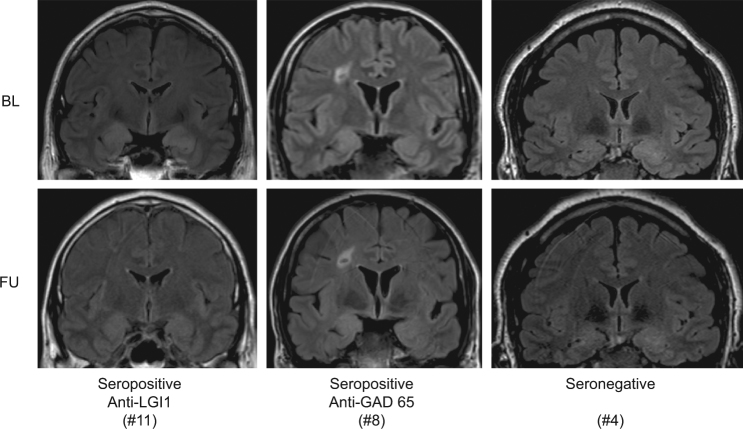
Representative FLAIR images at baseline (BL, upper panels) and follow-up (FU, lower panels) of confirmed autoimmune TLE-AE with anti-LGI1 aabs (Patient #11, left panels), anti-GAD65 aabs (Patient #8, middle panels), as well as suspected autoimmune TLE-AE (Patient #4, right panels) demonstrate uni- or bilateral hyperintensity and enlargement of the amygdala. Note that changes at BL were clearly regressive at FU in patient #11.
